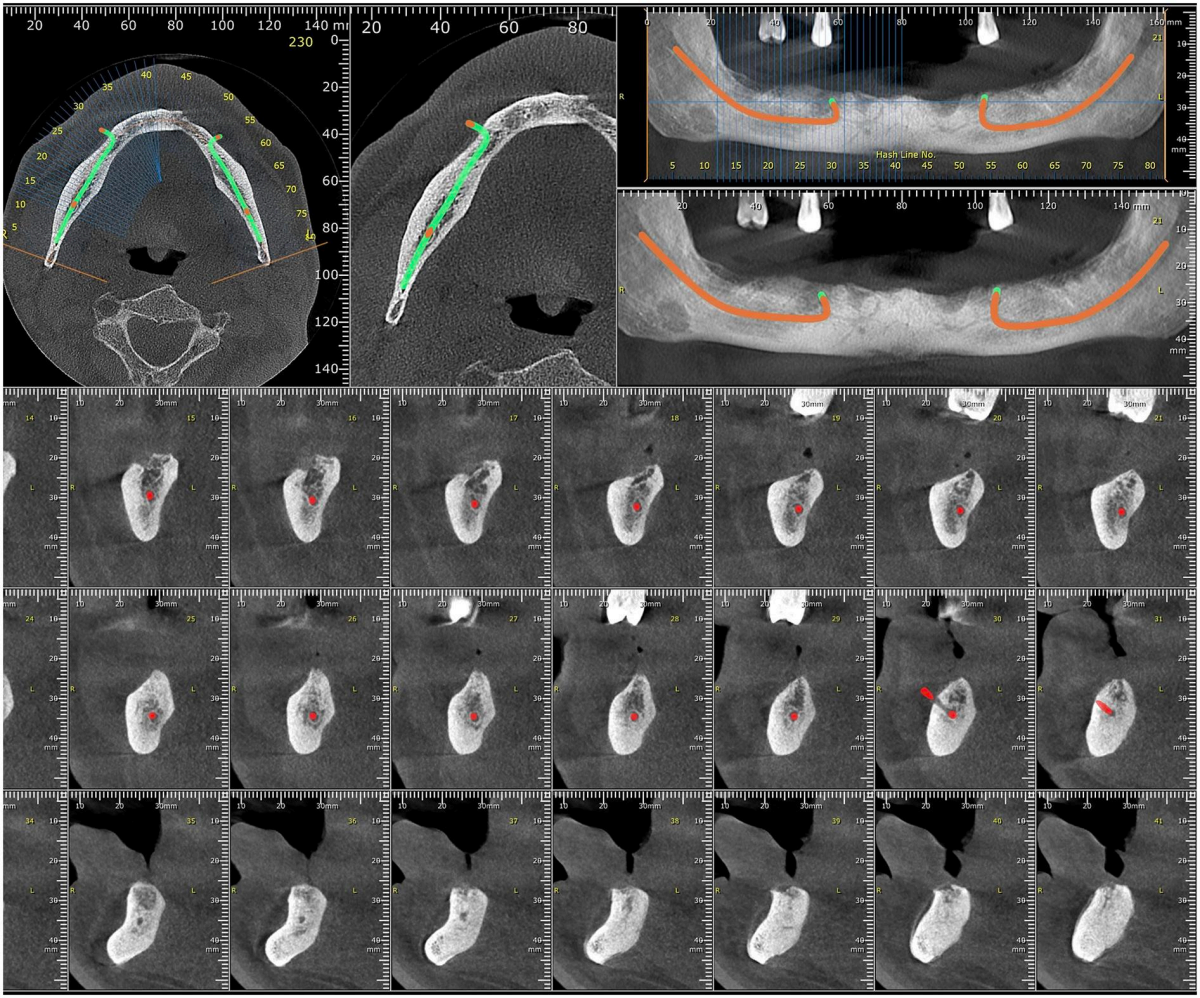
Cone-beam computed tomography (CBCT) axial and cross-sectional views showing absence of bone involvement and superficial course of the fistulous tract (not directly visualized due to soft tissue nature).
